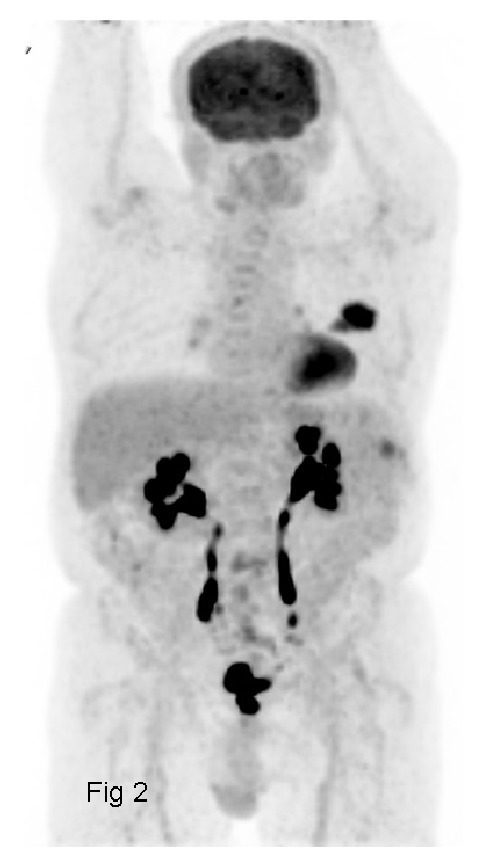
Whole-body 18FDG PET/CT image for 65-year-old man with papillary thyroid microcarcinoma reveals single hypermetabolic lytic mass in the fourth rib.
